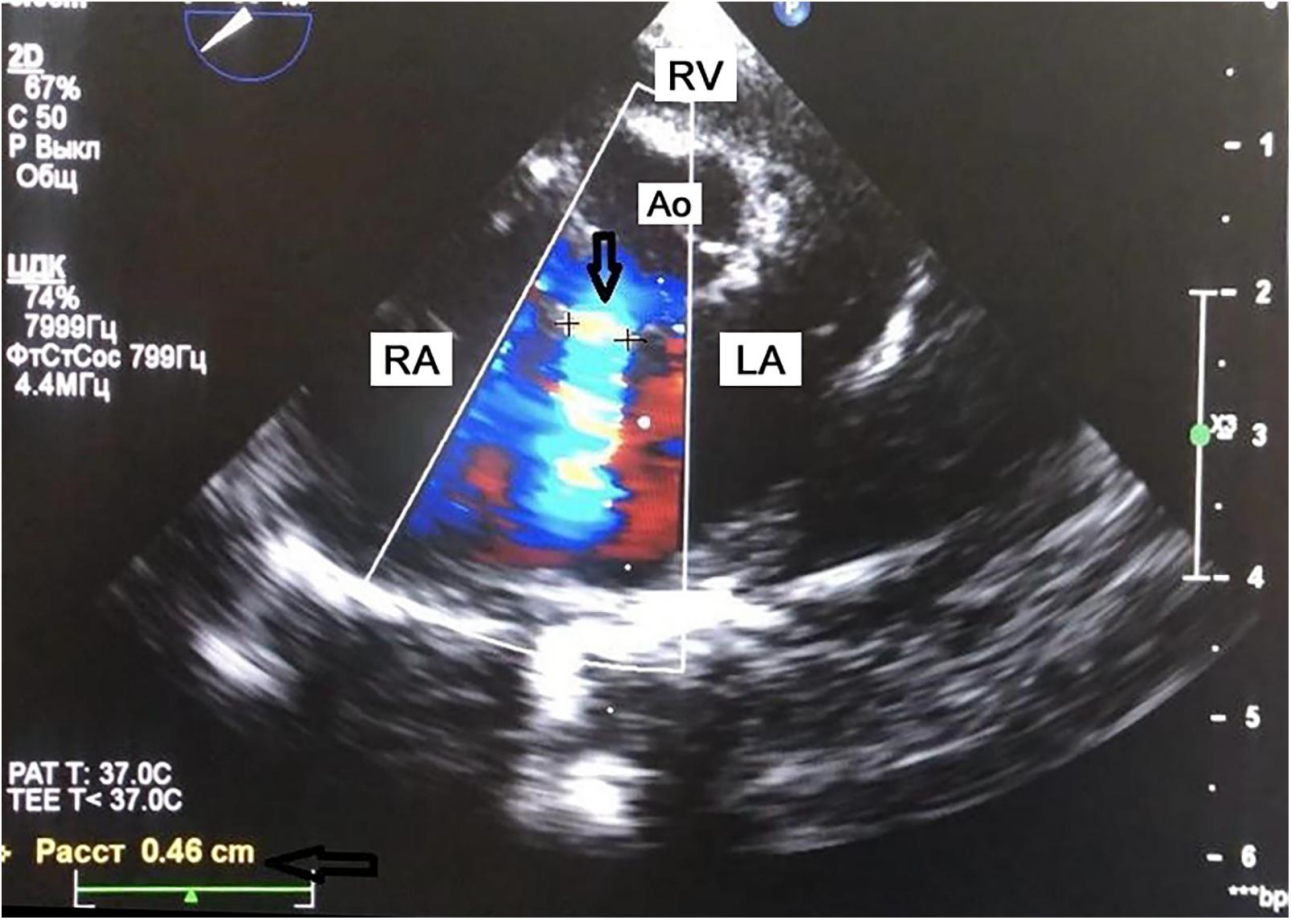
Short-axis view of ventricular septal defect via transesophageal echocardiography (TEE). The arrow points to the ventricular septal defect and shows its approximate size. In the lower left corner, the defect is visualized with a size of 0.46 cm. This is one of the positions used for the final selection of the type and size of the occluder. Traditionally, it is enough to use two positions: along the short axis (with an angle of 30–50 degrees) and along the long axis (with an angle of 120 degrees). This gives the maximum information about the defect in order to select the right occluder for it. RA, right atrium; Ao, aorta, RV, right ventricle; LA, left atrium.
